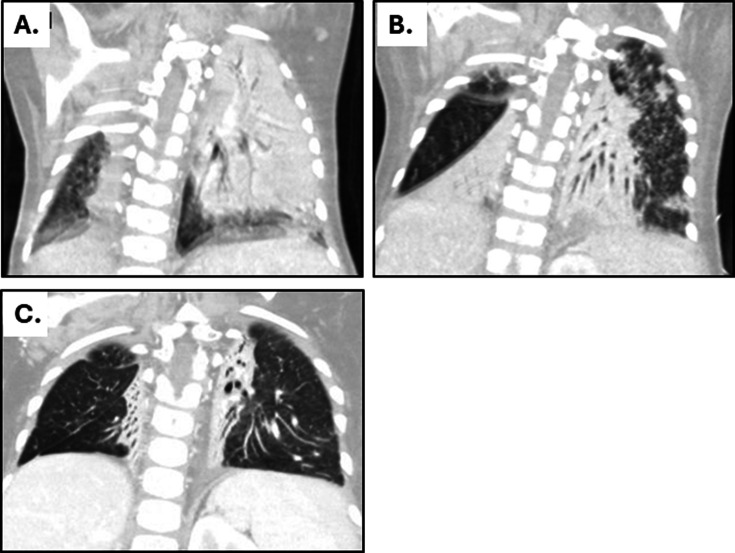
Case 2: chest CT with contrast. Images shown at (A) treatment initiation, (B) month 2 of therapy, and (C) month 20, end of treatment. CT images prior to the onset of *M. abscessus* infection are not available.
